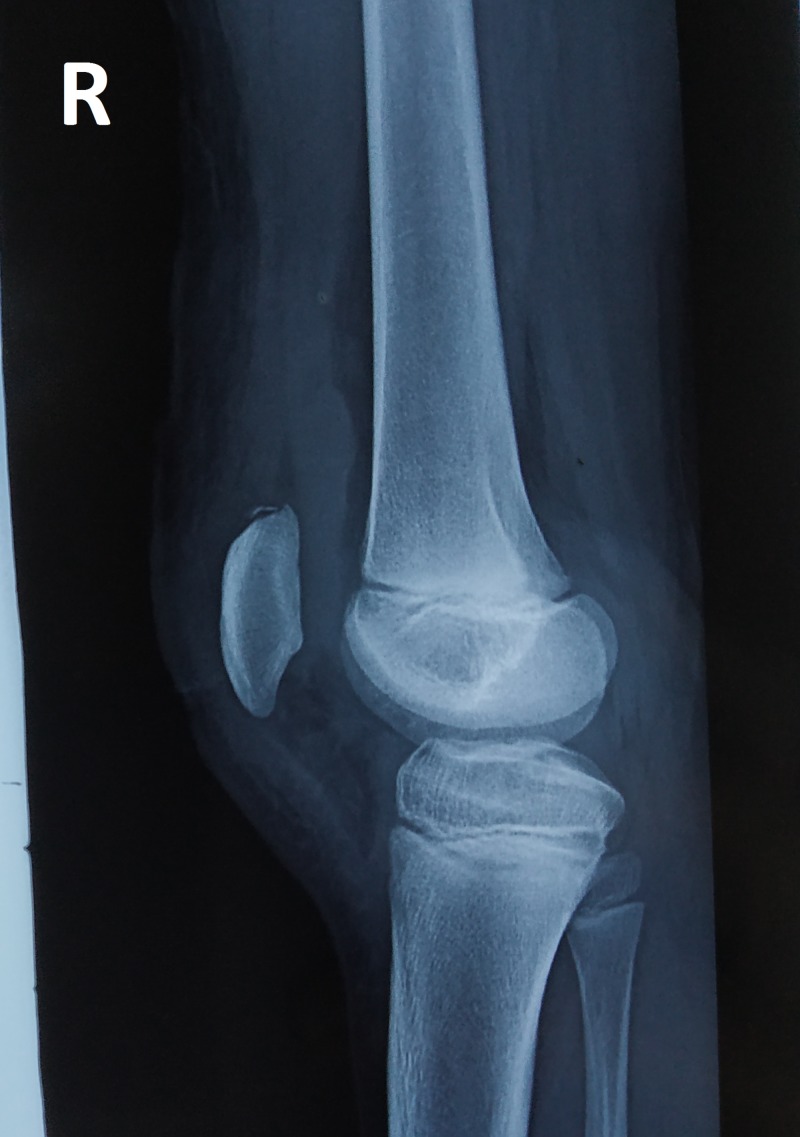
Post-operative X-ray of the right knee joint: lateral view
